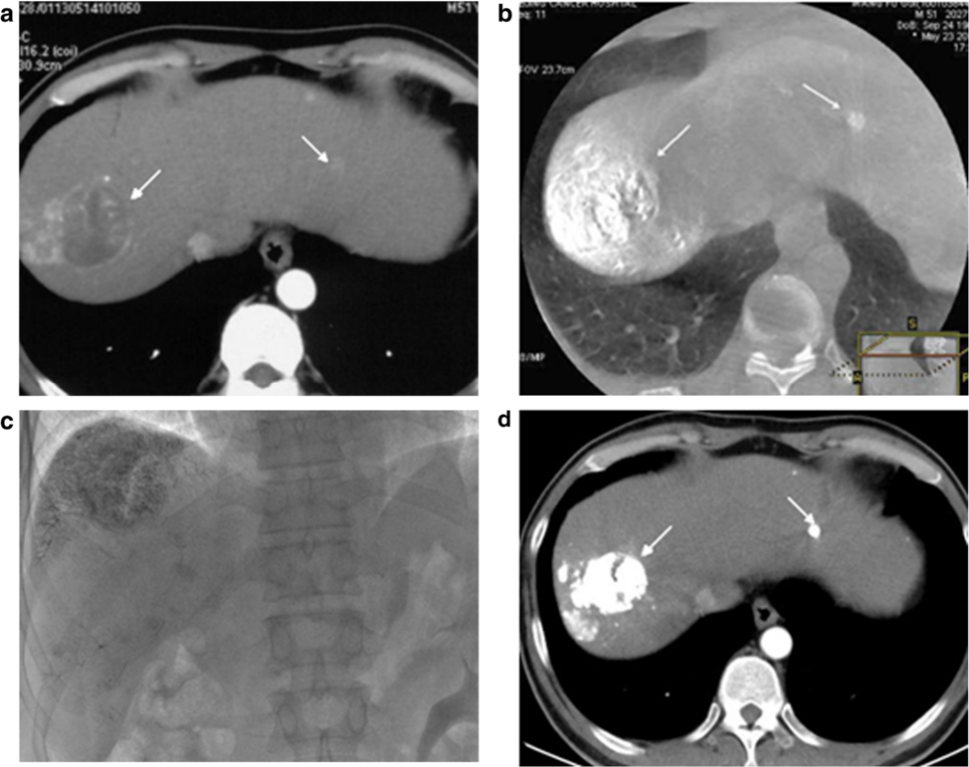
A 52-year-old male with multiple HCCs. a Preprocedure arterial phase contrast-enhanced CT shows index tumors in segments II and VII (*arrows*); b CBCT immediately after TACE showed these two tumor with complete lipiodol retention pattern concordance with CE-CT (*arrows*); c On the fluoroscopy image, complete lipiodol retention pattern is well shown on the large tumor in segment VII, but no retention in the small tumor in segment II. d Postprocedure 1 month follow-up contrast-enhancement CT shows both tumors without contrast enhancement (*arrows*) representing complete response
